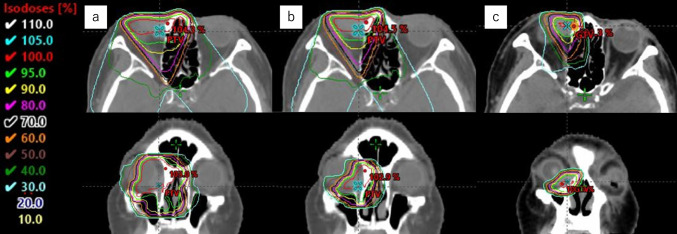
Radiation therapy uses 4 MV x-rays with 3-dimensional conformal radiation therapy (3D-CRT). The initial radiation field encompassed the primary tumor plus a 5-mm margin and the invaded ethmoid sinus. After delivering a dose of 40 Gy in 20 fractions, the radiation field was shrunken to the primary tumor plus a 2-mm margin for a dose of 60 Gy in 30 fractions, followed by a total boost of 70 Gy in 35 fractions to the primary tumor only to reduce the dose to the cornea. a 0–40 Gy. b 42–60 Gy. c 62–70 Gy
